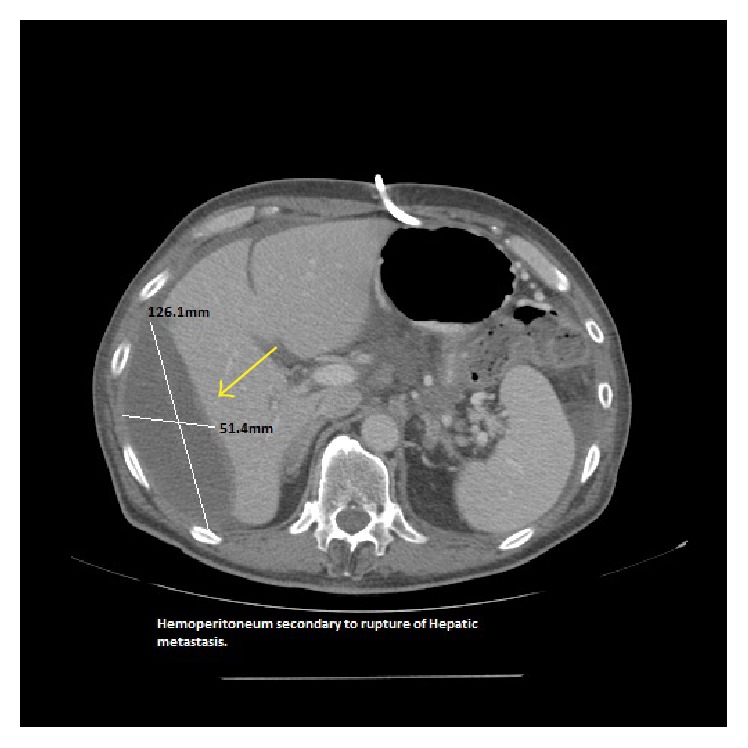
Axial contrast enhanced CT image (same study as in Figure 1) showing (yellow arrow) subcapsular liver collection.
